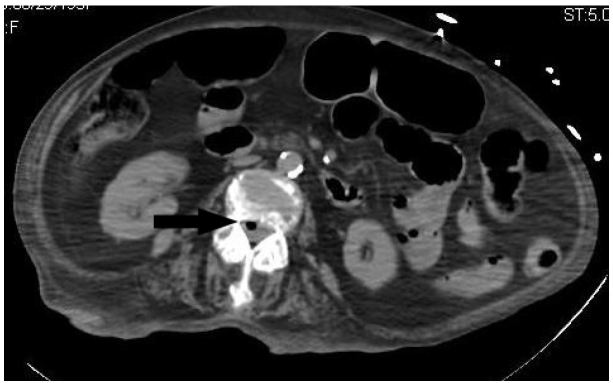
Computed tomography axial image of the abdomen demonstrating gas in the thoracic spinal canal (arrow).
